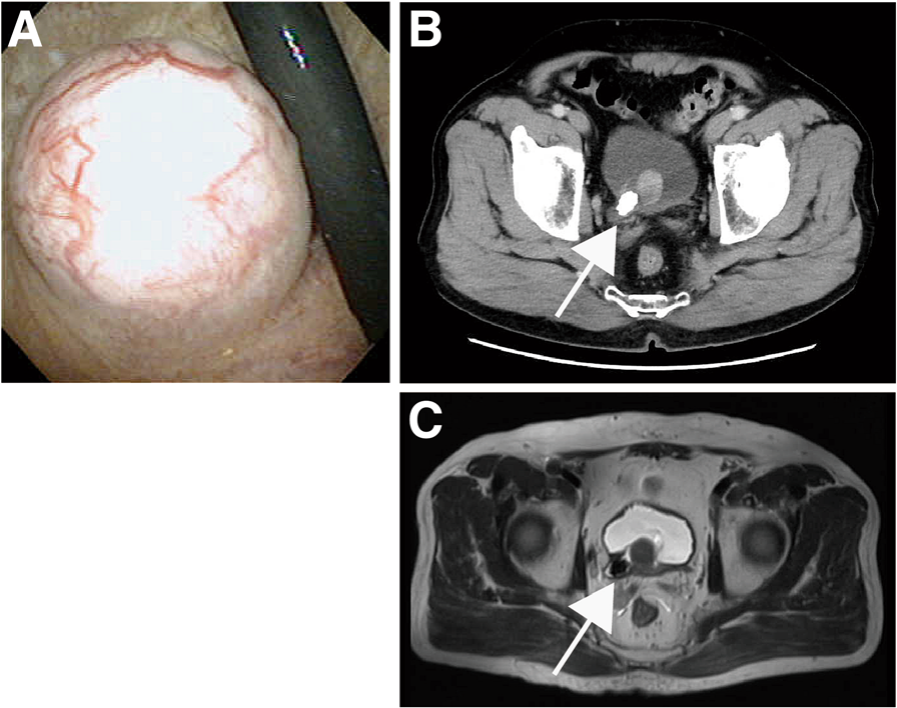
a Cystoscopy revealed a submucosal tumor covered with normal epithelium. b Enhanced computed tomography showed right ureteral calculus (*arrow*) and the tumor with enhancement. c Magnetic resonance imaging (T2-weighted image) revealed right ureteral calculus (*arrow*) and tumor
